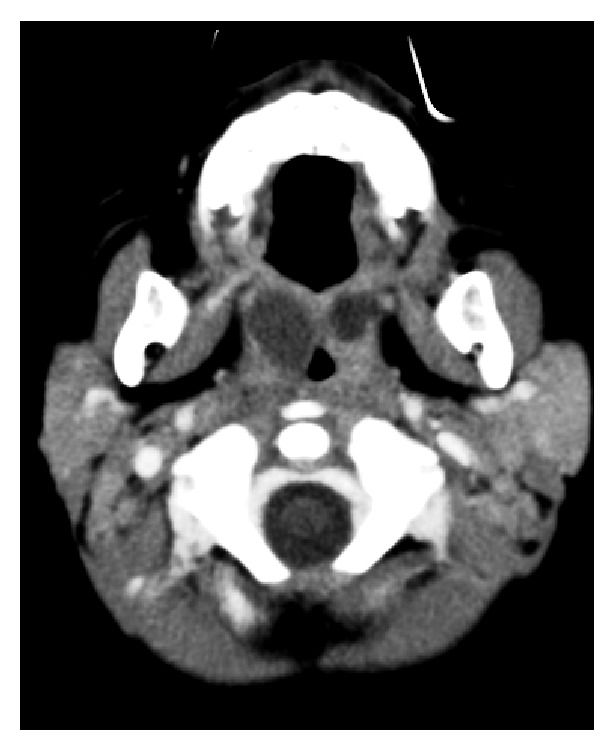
Contrast-enhanced computed tomographic scan, axial view. Enlargement of palatine tonsils and bilateral hypodense masses with thick rim enhancement. Slight obliteration of the parapharyngeal space.
